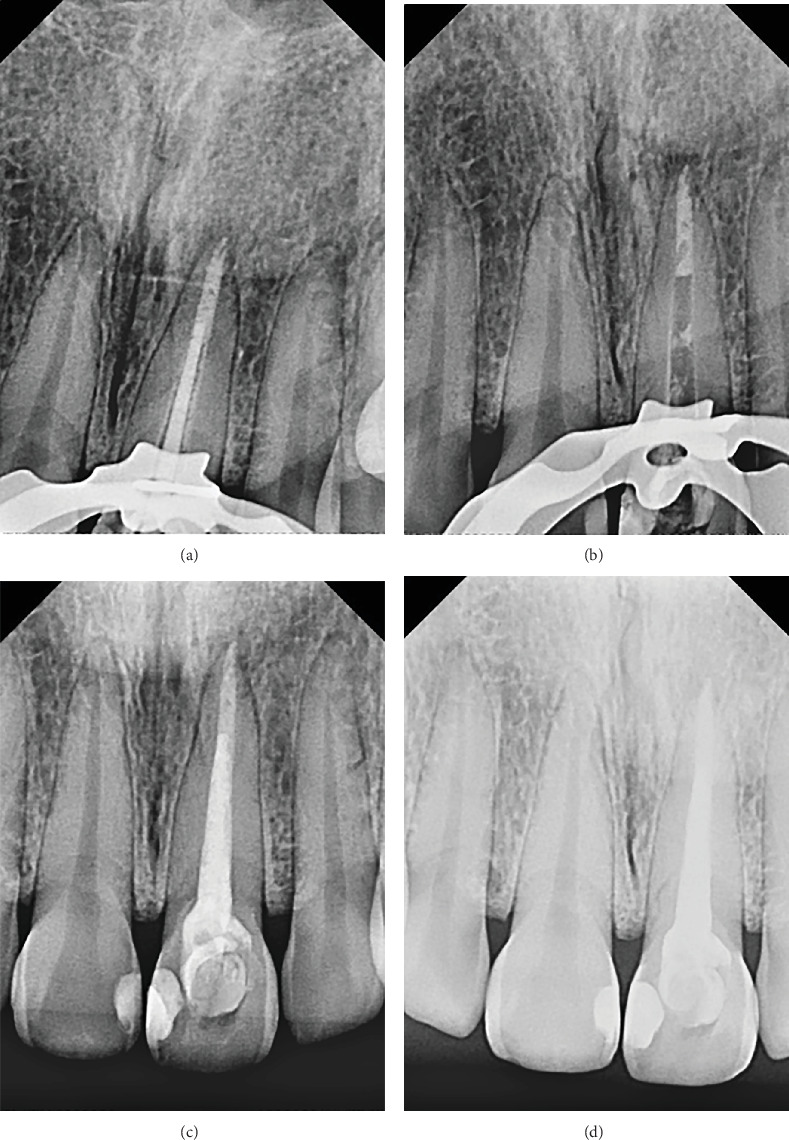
(a) Cone test. (b) MTA buffer. (c) Final radiograph. (d) One-month maintenance. Source: the author.
